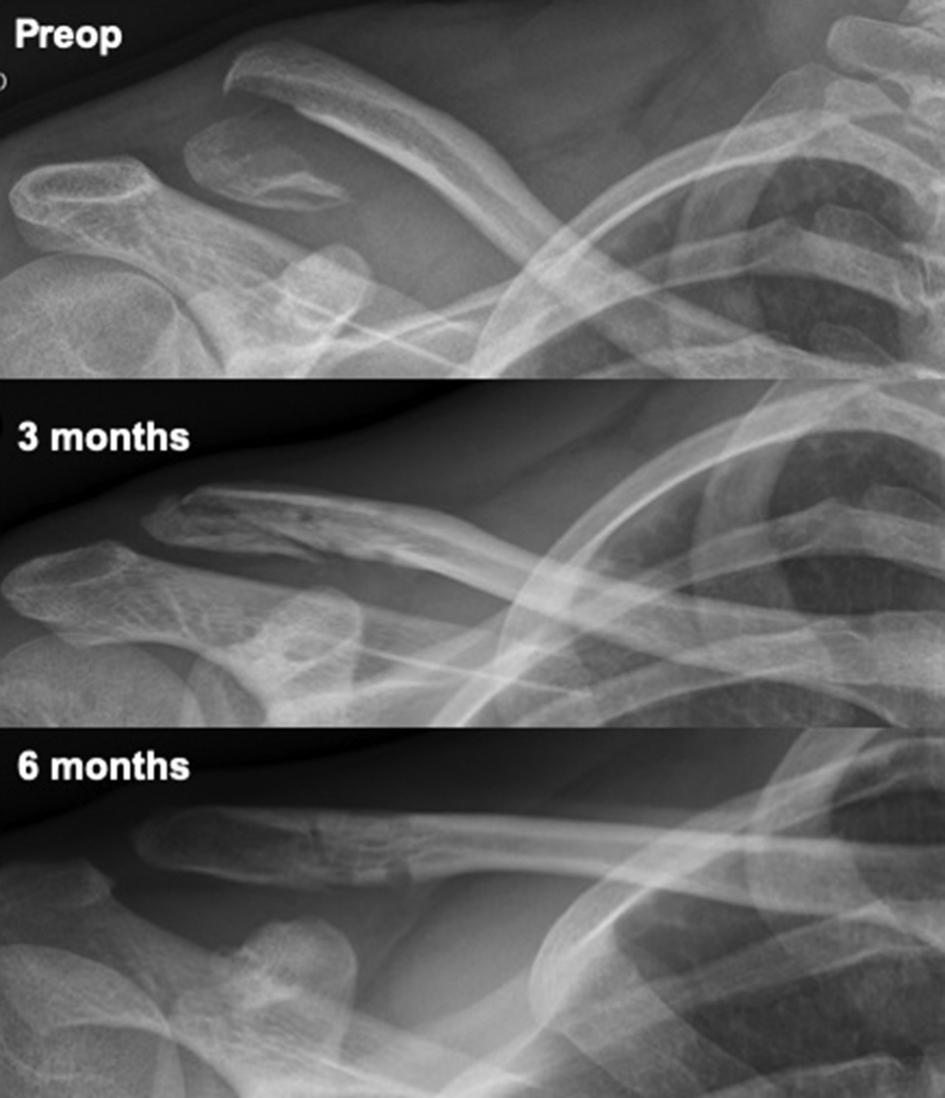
Radiographs showing a displaced Neer type II distal clavicle fracture, preoperatively and postoperatively at 3 and 6 months. Full union was achieved between 3 and 6 months.
